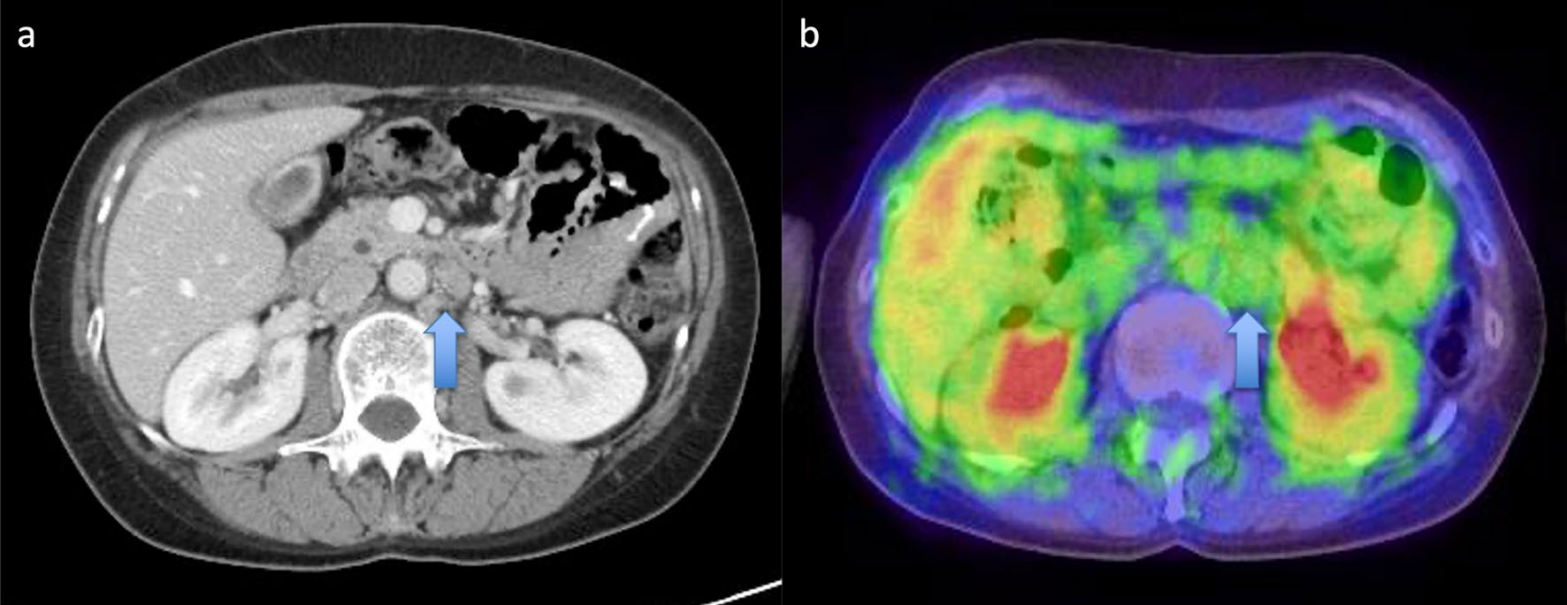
At postoperative year 5, computed tomography (a) /positron emission tomography (b) showed a 19 × 12 mm lymph node with no abnormal uptake in the No. 16b1lat lymph node station
